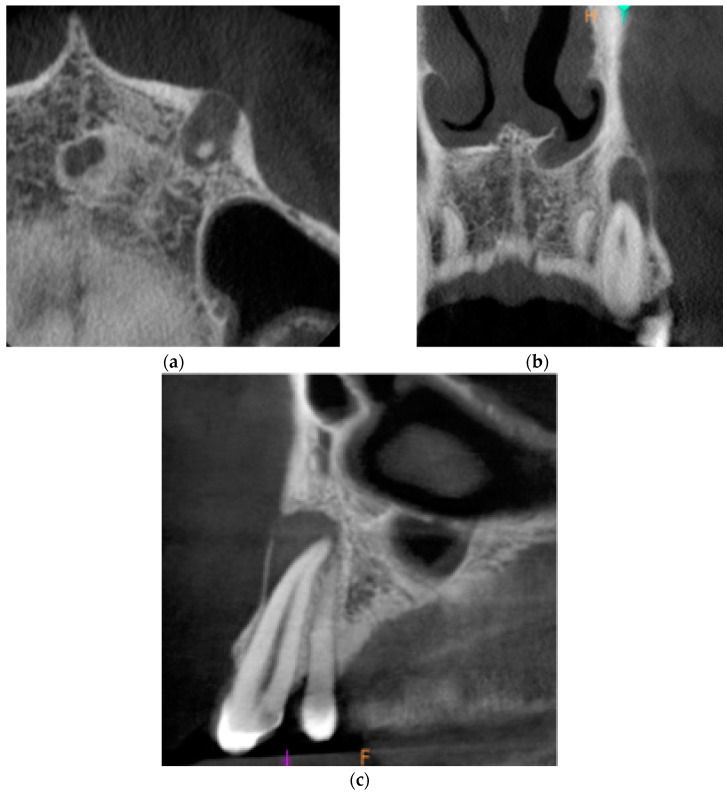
Preoperative CBCT images presenting the periapical lesion around the root of tooth no. 23 (date of CBCT examination: 19 January 2021): (a) axial view; (b) coronal view; and (c) sagittal view.
